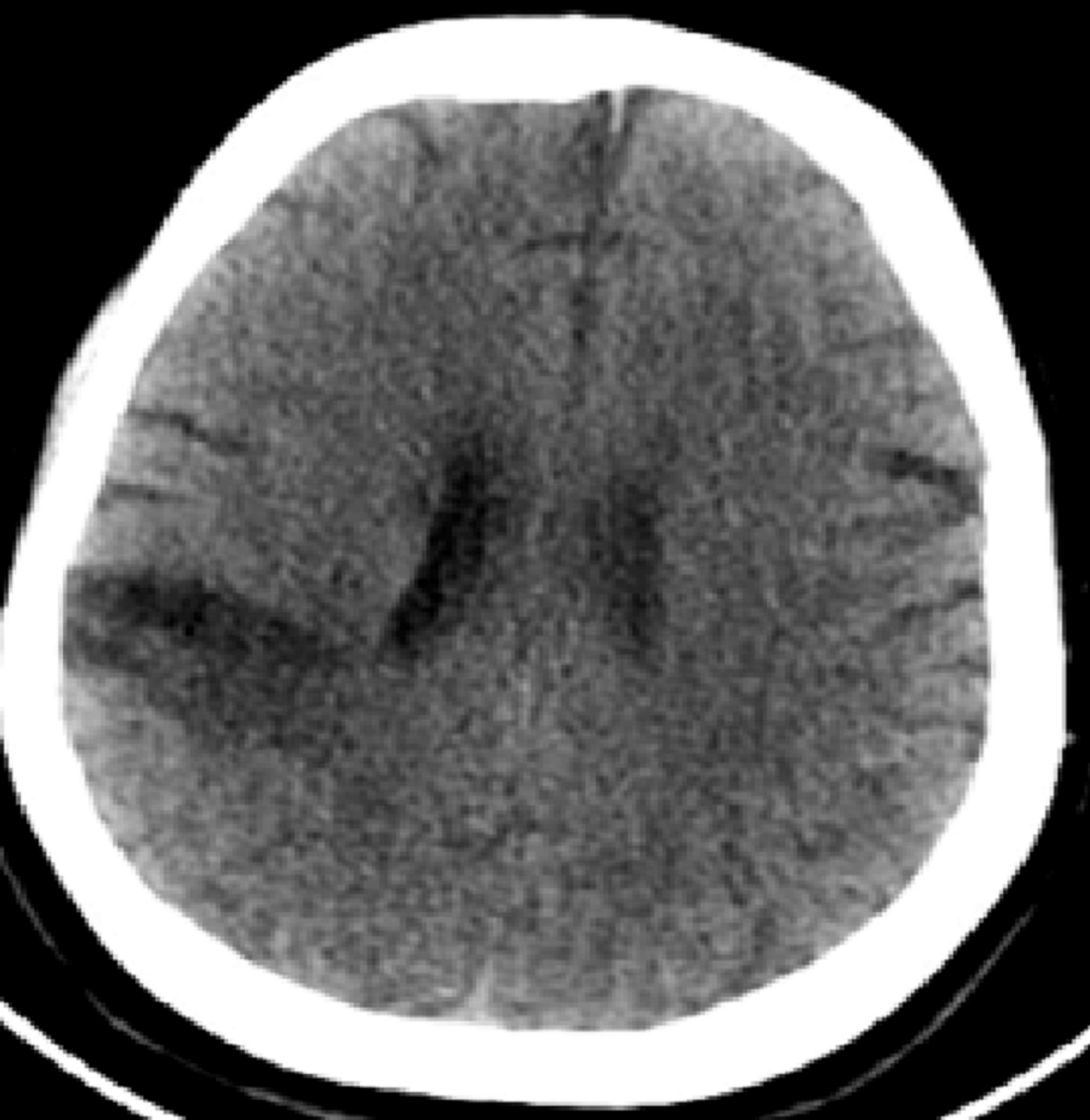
Cerebral CT scan: right posterior fronto-cortical subcortical hypodense area involving the territory of the right MCA, suggestive of late ischemic changes CT: computed tomography, MCA: middle cerebral artery
